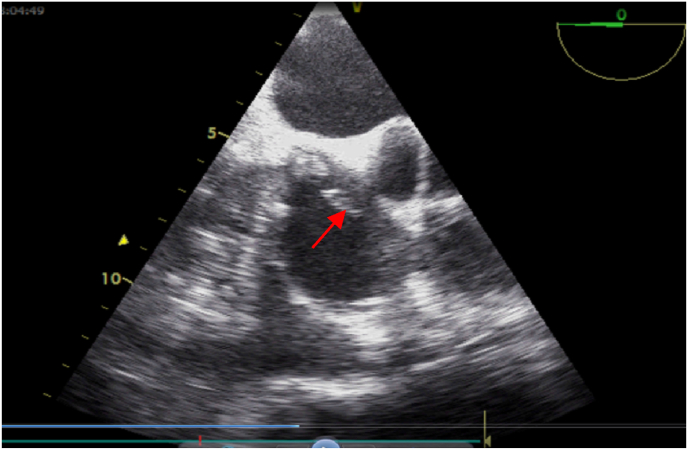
Transoesophageal echocardiography section showing vegetation at the haemodialysis catheter.
